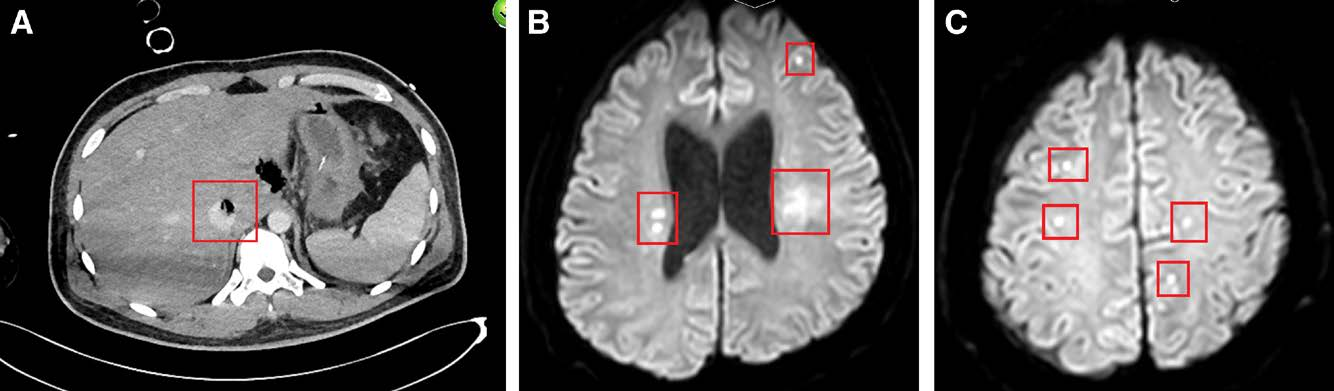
(A) Contrast-enhanced computed tomography (CT) of the chest, abdomen, and pelvis demonstrated patchy hypodense areas with gas formation in the caudate lobe of the liver and adjacent abdominal cavity, along with portal vein thrombosis. (B,C) Magnetic resonance imaging (MRI) of the brain revealed multiple abnormal signals in both cerebral hemispheres and bilateral ventricles.
